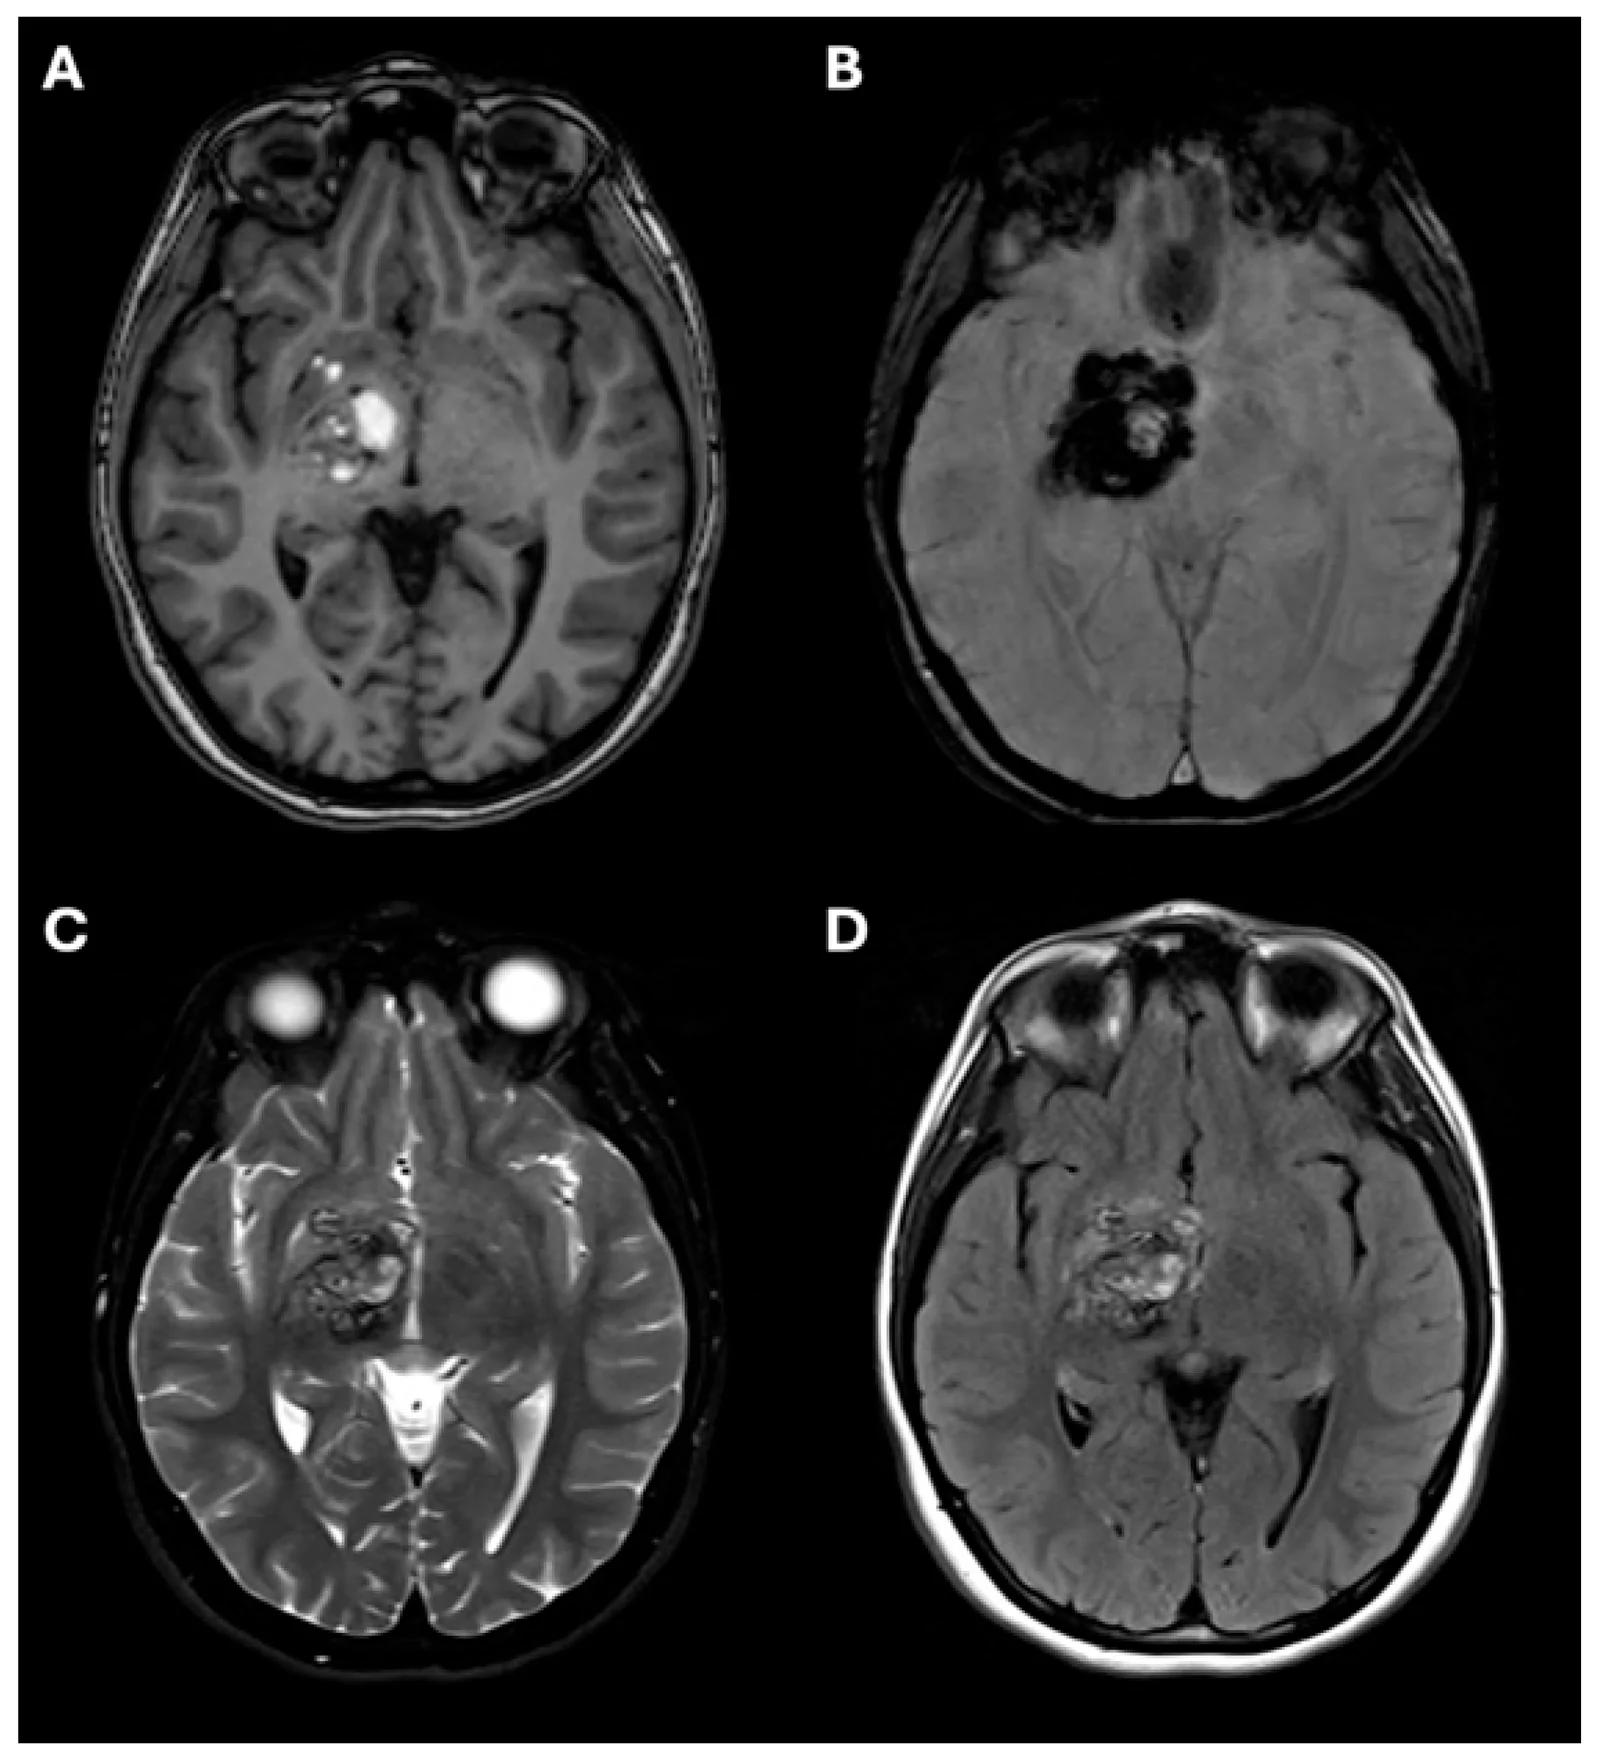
Brain MRI of a 13.5-year-old female with cerebral cavernous malformations (CCMs). Axial (A) T1-weighted image demonstrates intrinsic T1 hyperintensity; (B) susceptibility-weighted imaging (SWI) demonstrates prominent blooming artifact; and (C) T2-weighted and (D) T2-weighted FLAIR images demonstrate the characteristic heterogeneous “popcorn” appearance of CCMs.
